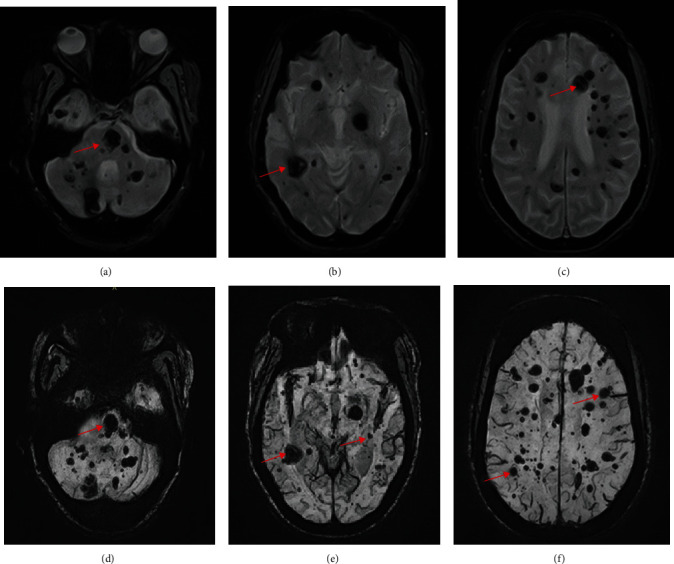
Axial brain MRI: (a–c) GRE/T2∗ and (d–f) SWI showing multiple diffuse cerebellar, pons, midbrain, bilateral basal ganglia, and cerebral lesions of varying sizes (red arrows) which became more prominent due to susceptibility artefacts (blooming) in keeping with multiple cerebral cavernomas.
